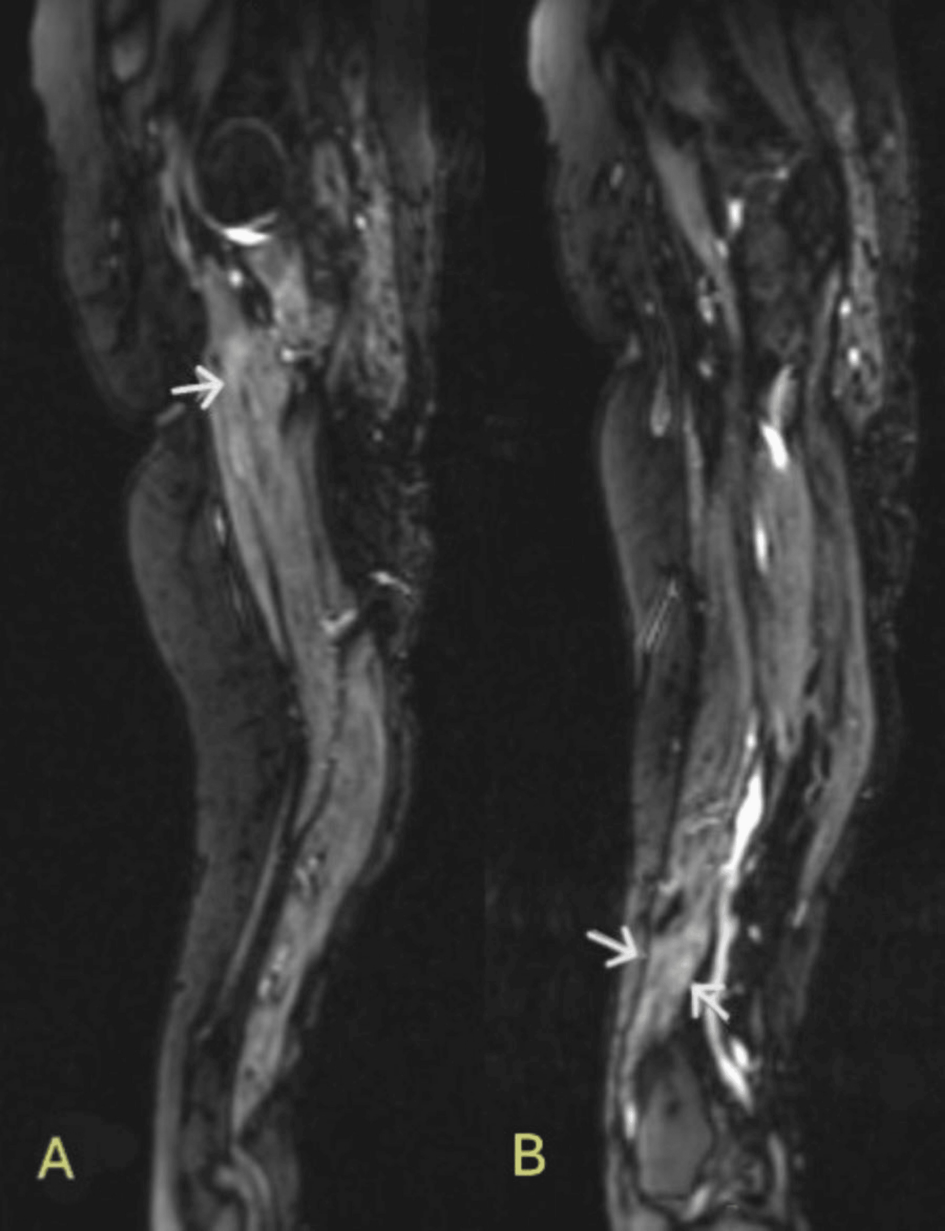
Bilateral MRI femur non contrast, T2 weighted sagittal images A: arrows point to fluid-sensitive enhancement around the adductor muscles. B: arrows point to fluid-sensitive enhancement around the vastus medialis muscles.
